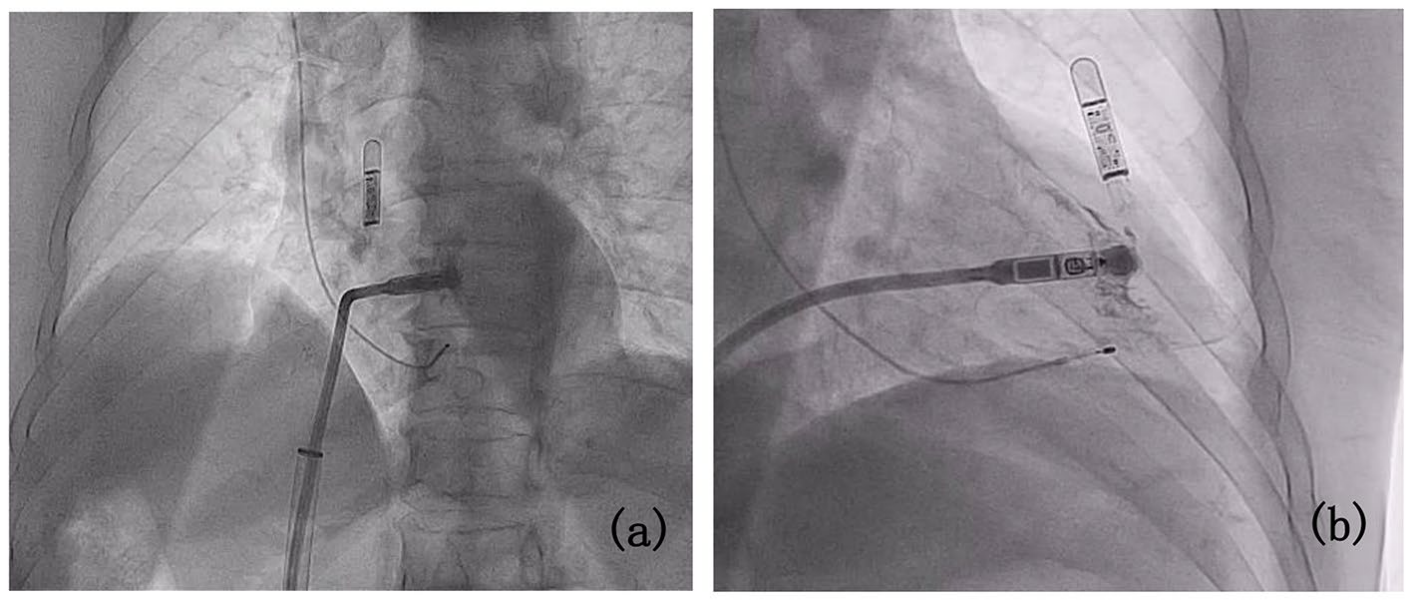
Post-implantation radiographs. Radiographs in the left and right anterior oblique projections (a and b) following implantation of the leadless pacemaker
